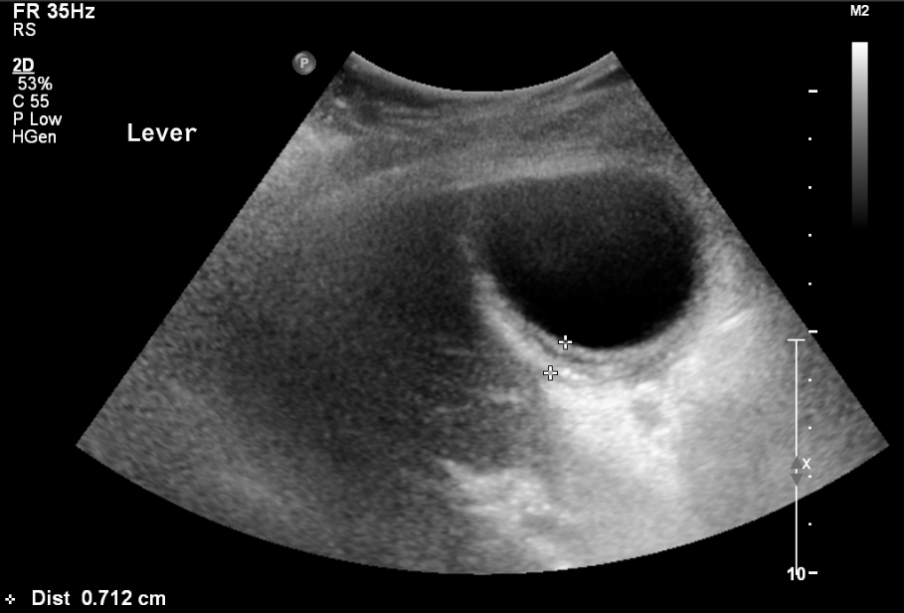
Ultrasound image of the gallbladder. Ultrasound image of the gallbladder acquired at presentation of the patient in the emergency department. The gallbladder is shown with a thickened wall (7 mm) suggesting inflammation.
